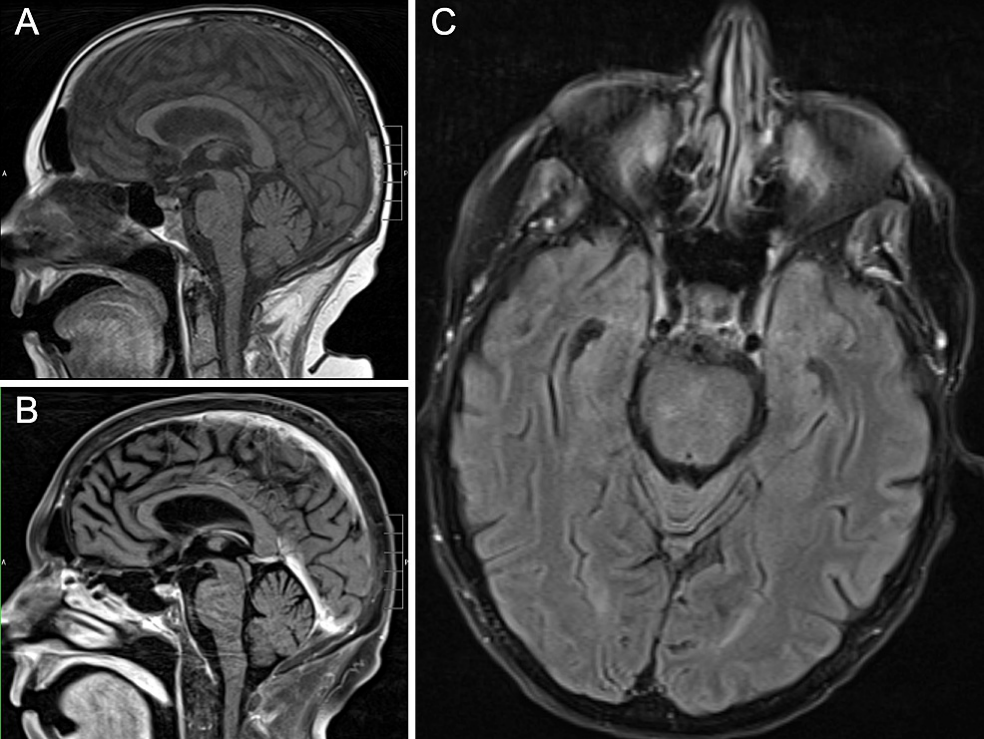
MRI brain Brain MRI was performed to evaluate encephalopathy that developed during the hospital course. Representative sagittal views (A, T1 FLAIR and B, T2 fluid-attenuated inversion recovery (FLAIR)) and transverse view (C, T2 FLAIR) showed no evidence of meningitis, encephalitis, mass lesions, hydrocephalus, acute ischemia, or hemorrhage.
